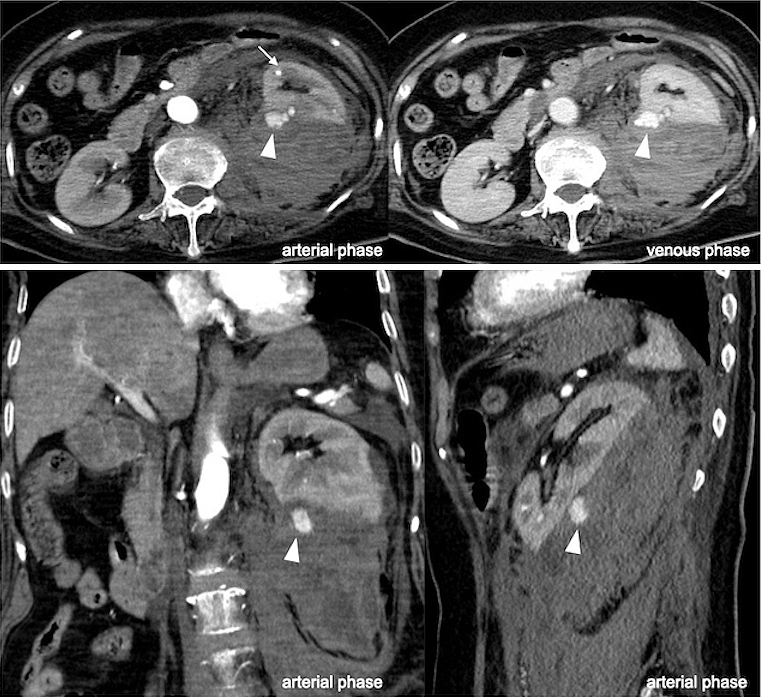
Images of computed tomography with abdominal hematoma. Contrast medium extravasation from the kidney (arrowhead) and aneurysm formation (arrow).
